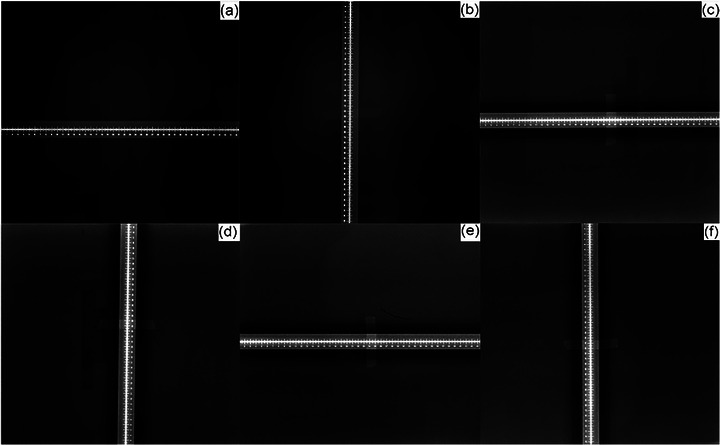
Radiographic images of effective imaging area. Radiopaque ruler measurements for CBCT image in (a) x‐axis and (b) y‐axis; radiopaque ruler measurements for DR1 image in (c) x‐axis and (d) y‐axis; radiopaque ruler measurements for DR2 image in (e) x‐axis and (f) y‐axis. DR1 and DR2 are the flat panel detectors of the dual‐panel DR system. DR, digital radiography; CBCT, cone‐beam computed tomography.
